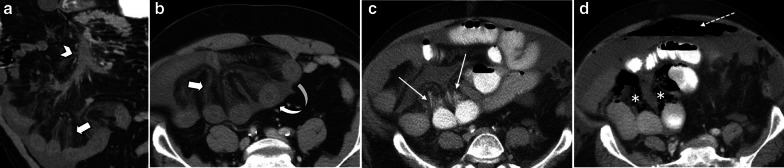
Progression of venous ischemia from early to late stage in a 58 y/o male presenting with abdominal pain. On day 1 (a, b), baseline Imaging demonstrates extensive SMV thrombosis (arrowhead) with associated small bowel wall (curved arrow) and mesenteric edema (thick arrow), compatible with early stage of ischemia. On day 3 (c), follow-up scan demonstrates bowel wall thinning with extraluminal oral contrast (thin arrows) as mesenteric venous contrast intravasation, suggestive of Intermediate stage ischemia. On day 7 (d), subsequent CT shows extensive pneumatosis (asterisks) and pneumoperitoneum (dotted arrow), in keeping with late-stage ischemia. Please notice that oral contrast intravasation can precede transmural bowel necrosis
